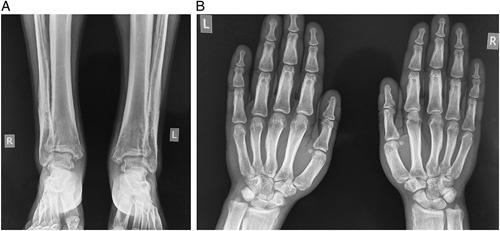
(A) Radiograph of ankle joint showing periosteal bone formation and cortical thickening. (B) Radiograph of bilateral hands showing hyperostosis of metacarpals, proximal, and middle phalanges.
